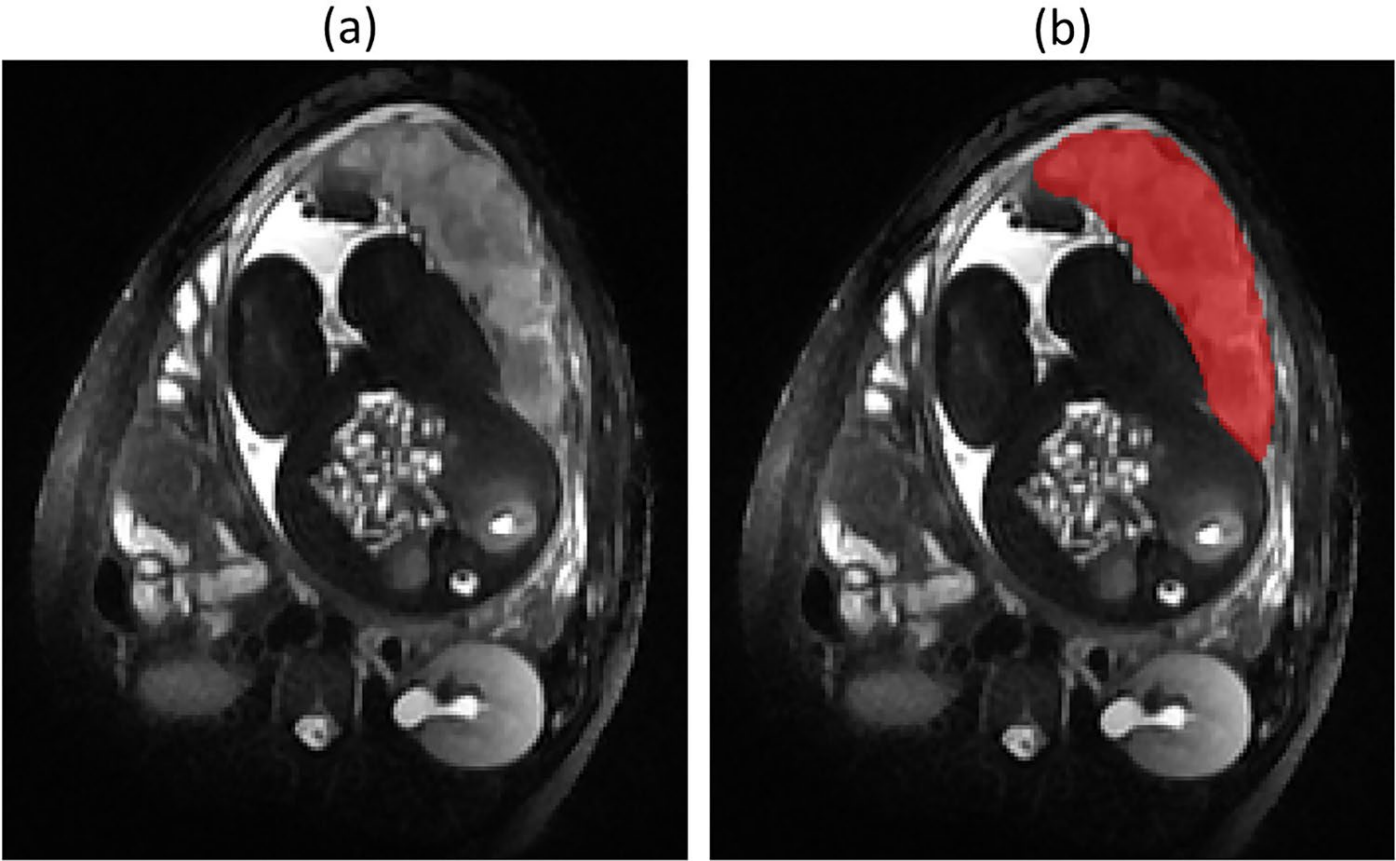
Image segmentation of a representative placenta. (a) T2-weighted placenta image; (b) Manual segmentation of the placenta.
